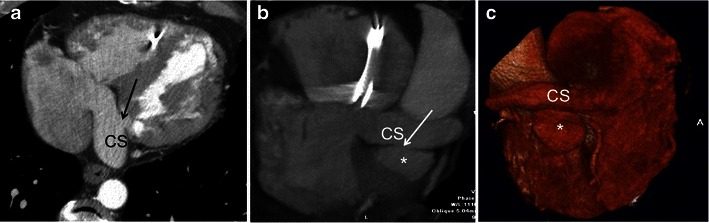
A 50-year-old male with repaired tetralogy of Fallot and CS diverticulum. He has a pacemaker. a Axial CT, b maximum intensity projection and c volume-rendered CCT images demonstrate a congenital diverticulum (b, c, asterisks) arising from the CS, with a narrow neck (a, b, arrow). The diverticulum lies between the CS and the mitral valve annulus. The CS is enlarged, which could be a source of arrhythmia
